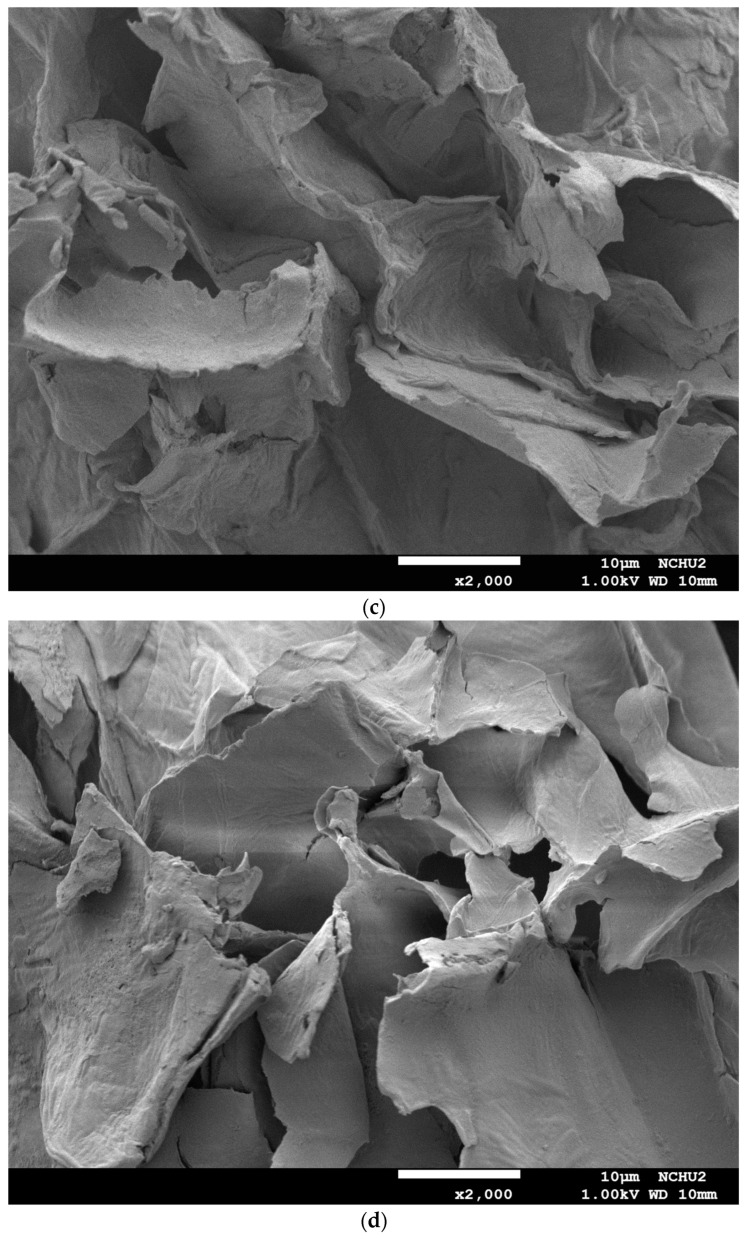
Comparison of FESEM images of dried burdock roots before and after extraction at 50 °C, 1/30 (g/mL-water) of solid-to-liquid ratio, and 10 min of extraction time: (a) dried burdock root powder before extraction (×2000); (b) extraction residue using 120 rpm of shaking (×2000); (c) extraction residue using 40 kHz of ultrasound (×2000); (d) extraction residue using 120 kHz of ultrasound (×2000).
